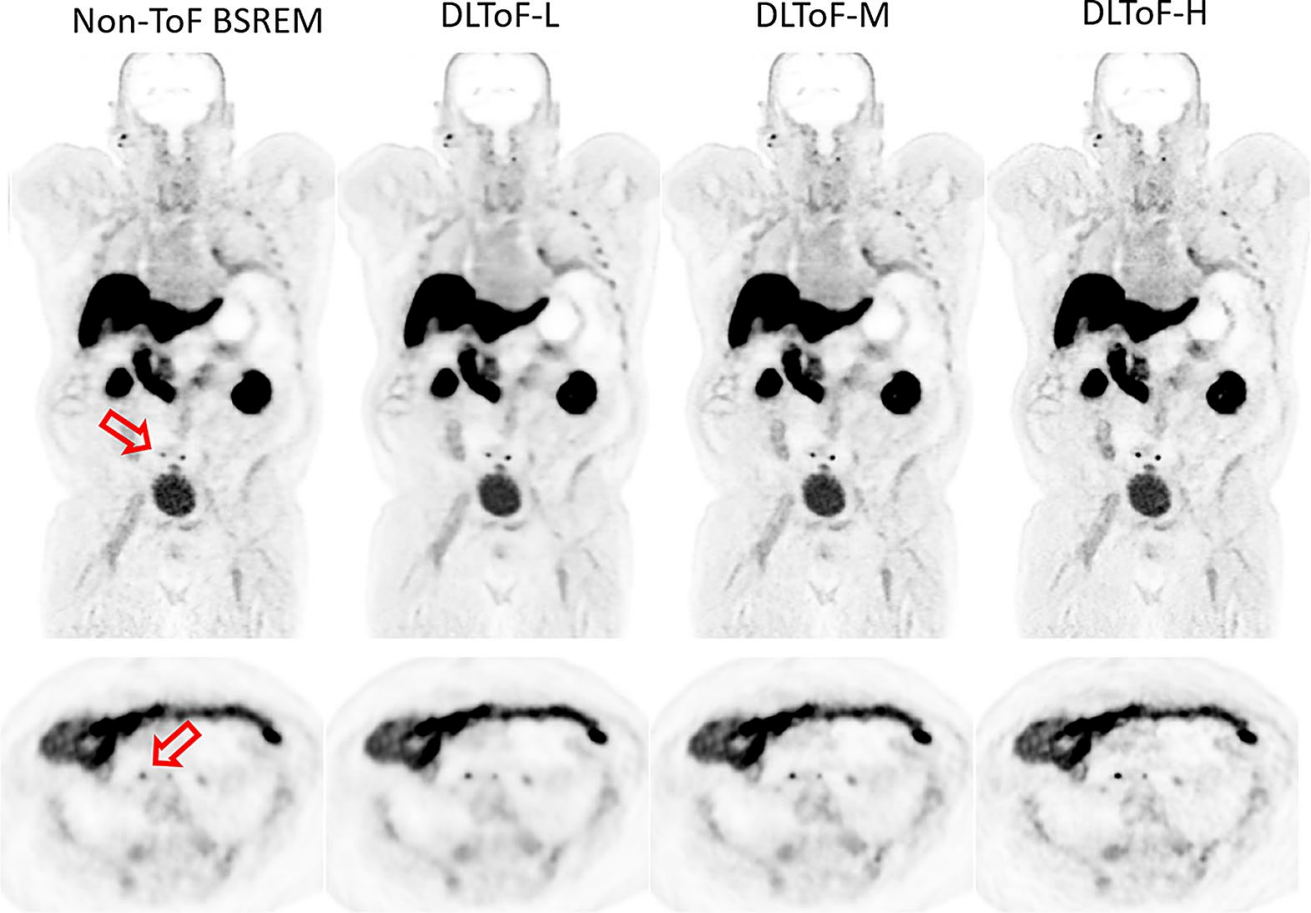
DL-ToF enhancement of a representative ^18^F-PSMA-1007 test subject with a BMI of 39.1 kg/m^2^ with an injected activity of 249 MBq scanned on a GE Omni Legend™ PET/CT scanner. Demonstrating two sub-5 mm PSMA avid retroperitoneal nodes at the L5 level. Display window: 0–6 SUV
